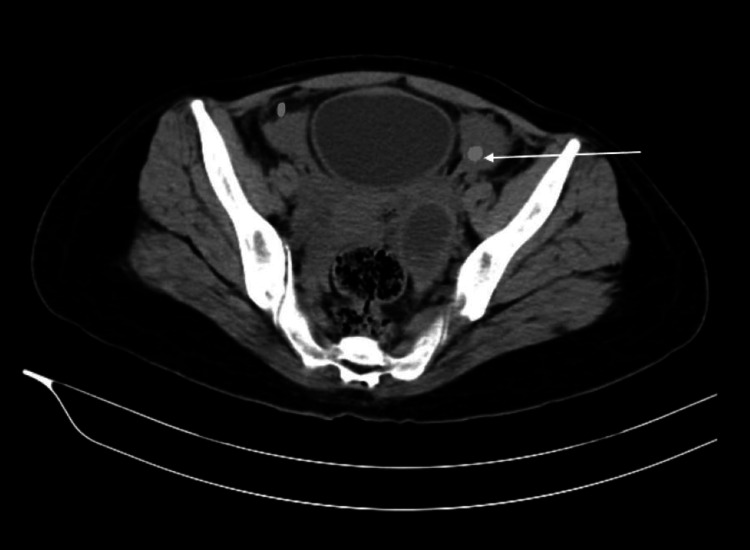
Contrast-enhanced computed tomography of the abdomen and pelvis of the patient at presentation. In the left ovary: a hemorrhagic cyst measuring 4.3 x 3.5 x 5.1 cm was found
